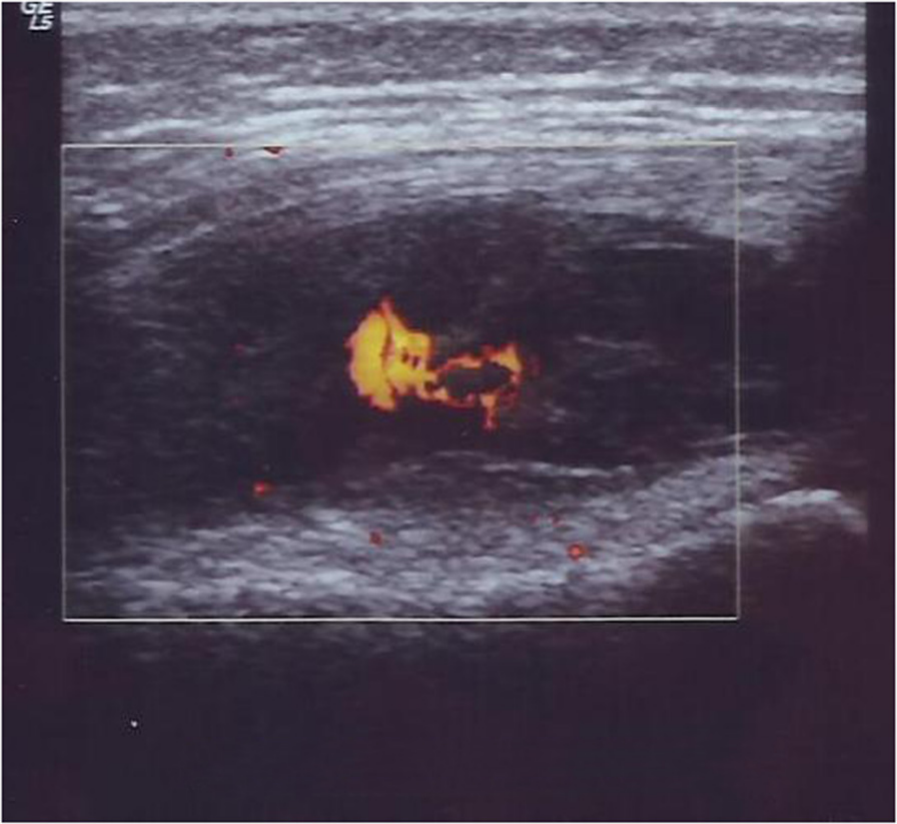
Case 1 left knee ultrasound. Transverse gray-scale and color Doppler 12–5 MHz ultrasound image obtained over the medial aspect of the knee showing abundant synovial pannus filling the subquadriceps recess as a band of hypoechoic tissue intermingled with fluid. Marked synovial hyperemia is observed at color Doppler examination indicating active pannus.
